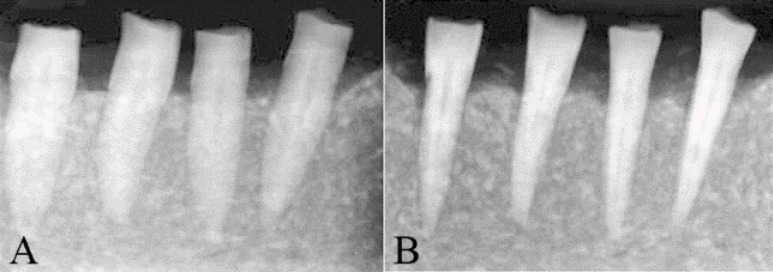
A)* Angled and *B)* straight radiographies
